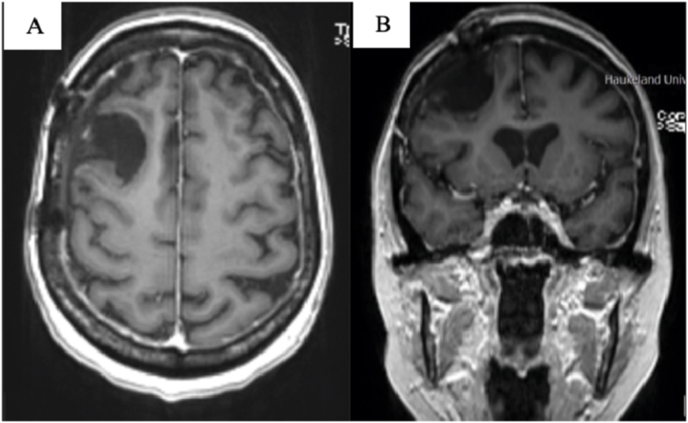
Contrast-enhanced T1-weighted images after surgery. A: MRI in axial plane. B: MRI in coronal plane.
